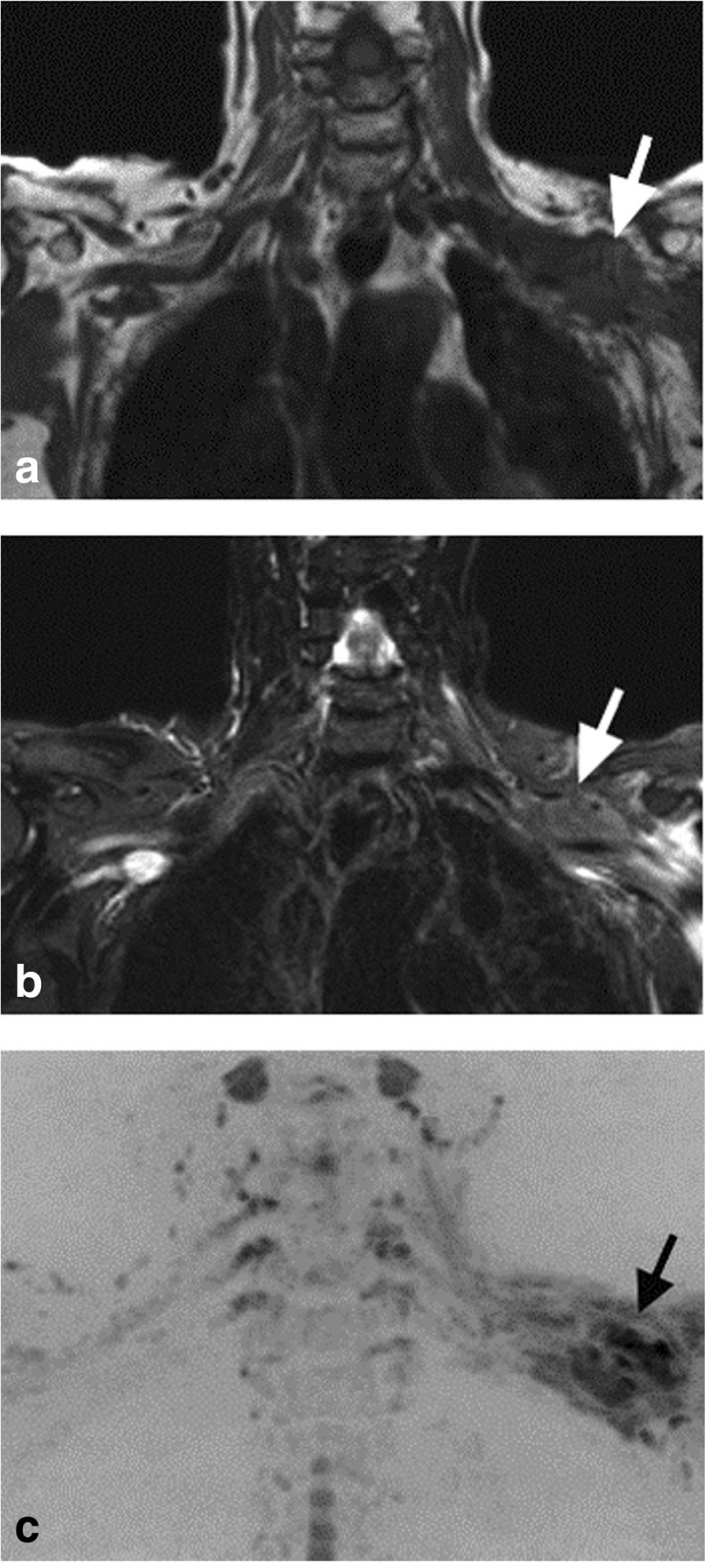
A 38 year-old women with history of left breast cancer presenting with left arm pain. (a) Coronal T1-weighted, (b) coronal STIR and (c) coronal inverted greyscale maximum intensity projection of the b = 700 s/mm^2^ images. Scans demonstrate irregular thickening at the level of the cord and divisions of the left brachial plexus, appearing of low T1-signal intensity, intermediate to high STIR signal intensity, and shows low signal intensity impeded diffusion (arrows). The disease in this case, was scored “5” on both conventional imaging and on the combination of conventional imaging and DW-MRN.
